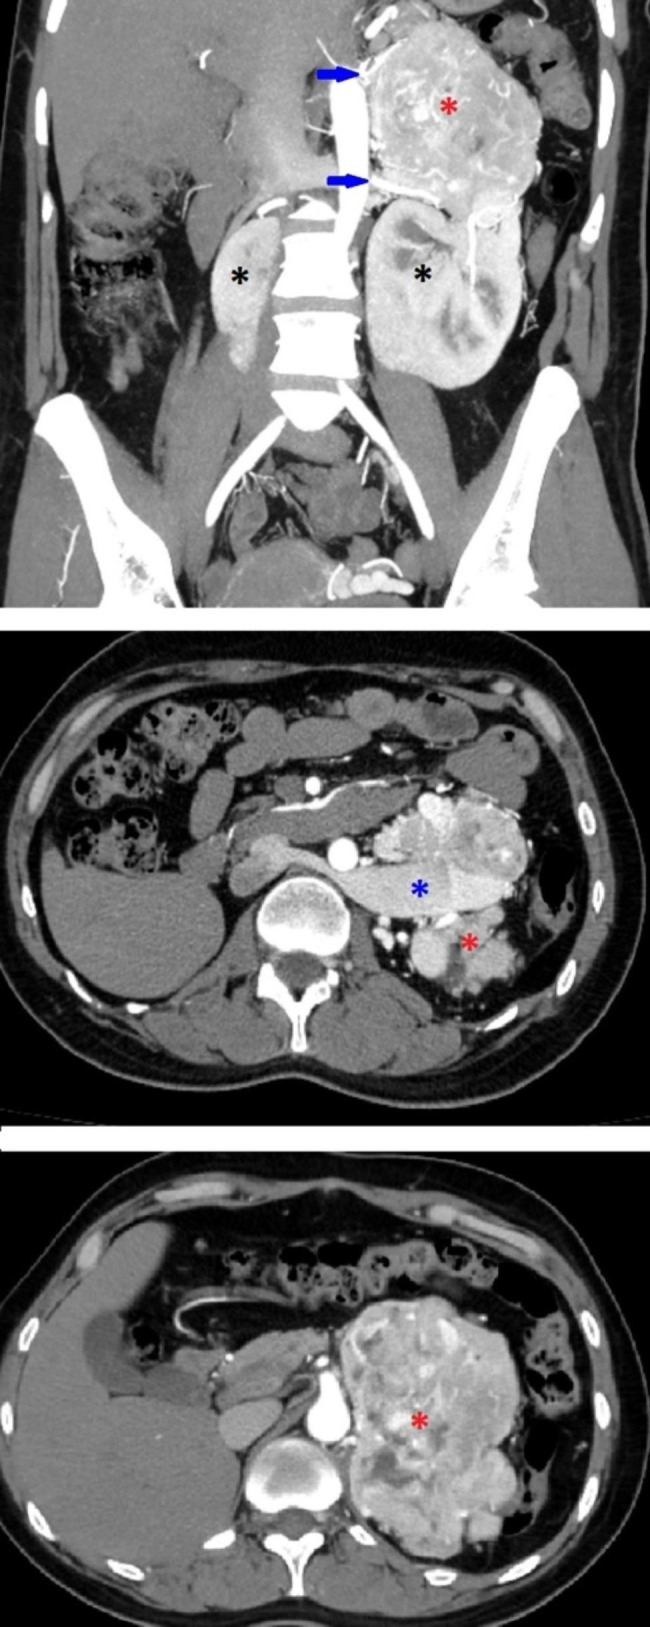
The preoperative CT angiogram in the coronal (A.) and axial (B., C.) planes shows a large left adrenal mass (red asterisk), pelvic horseshoe kidney (black asterisks), severely dilated retro-aortic renal vein (blue asterisk) and several large aortic branches supplying the giant adrenal mass (blue arrows)
